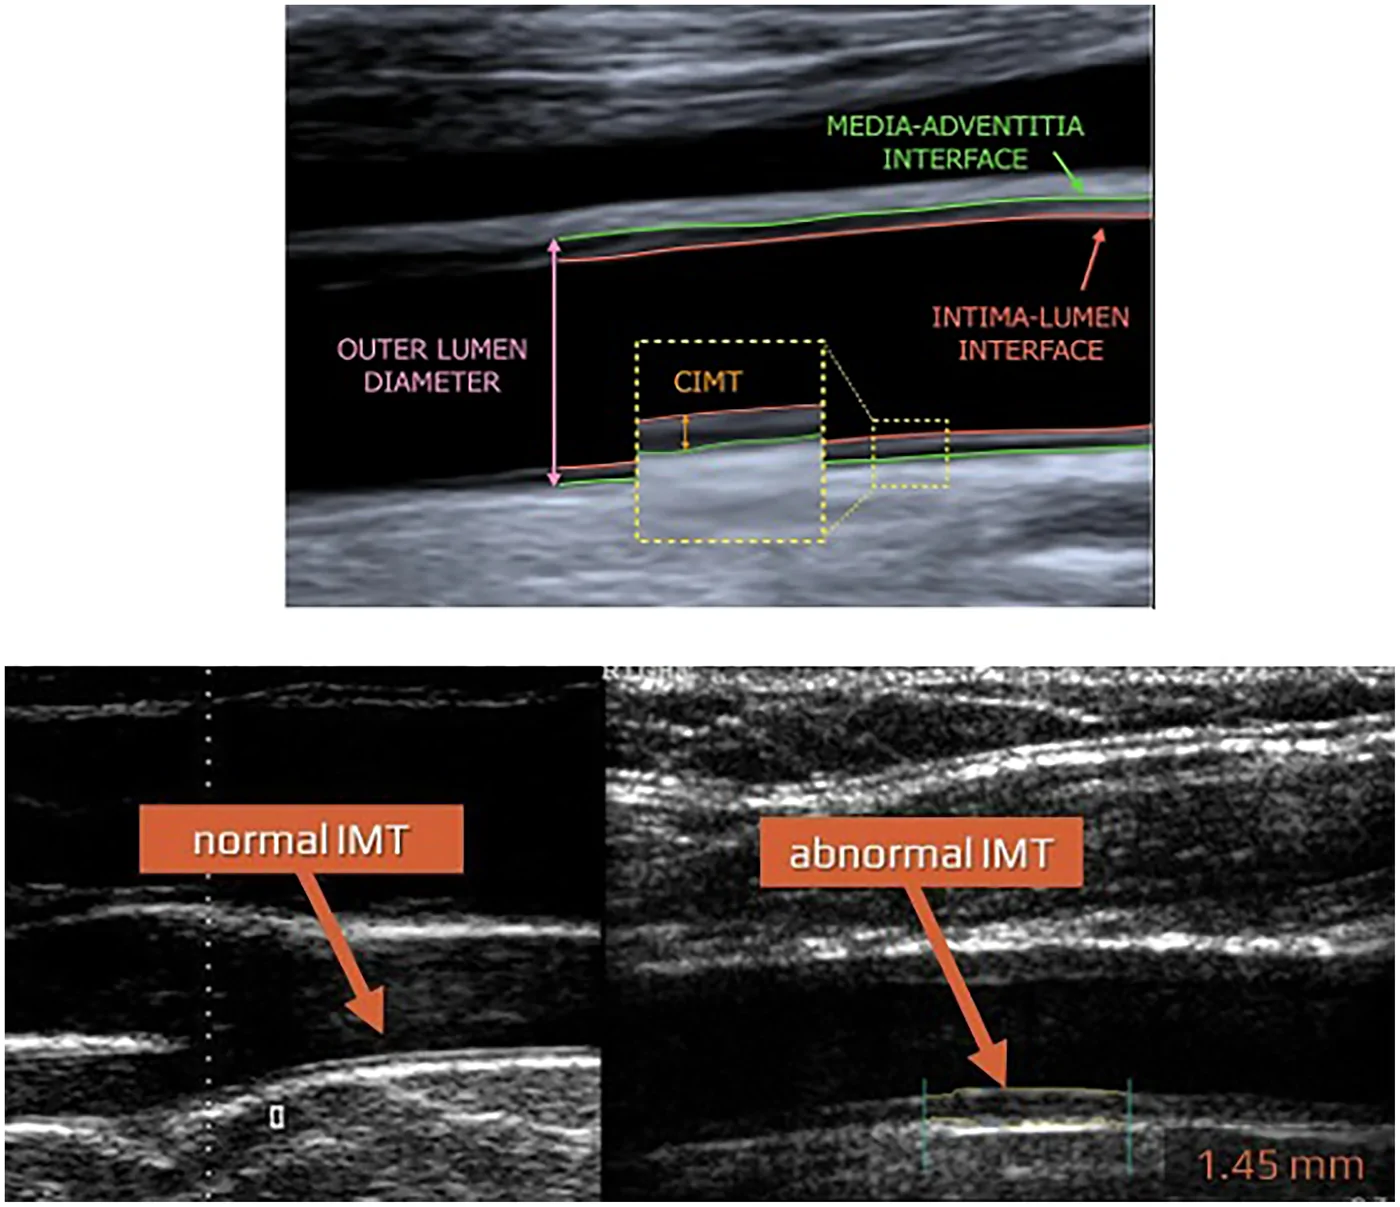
Measurement of carotid intima-media thickness (cIMT). High-resolution B-mode ultrasonography demonstrating measurement of the far wall of the distal common carotid artery approximately 1 cm proximal to the carotid bulb. Three measurements were obtained from each carotid artery and averaged for analysis.
